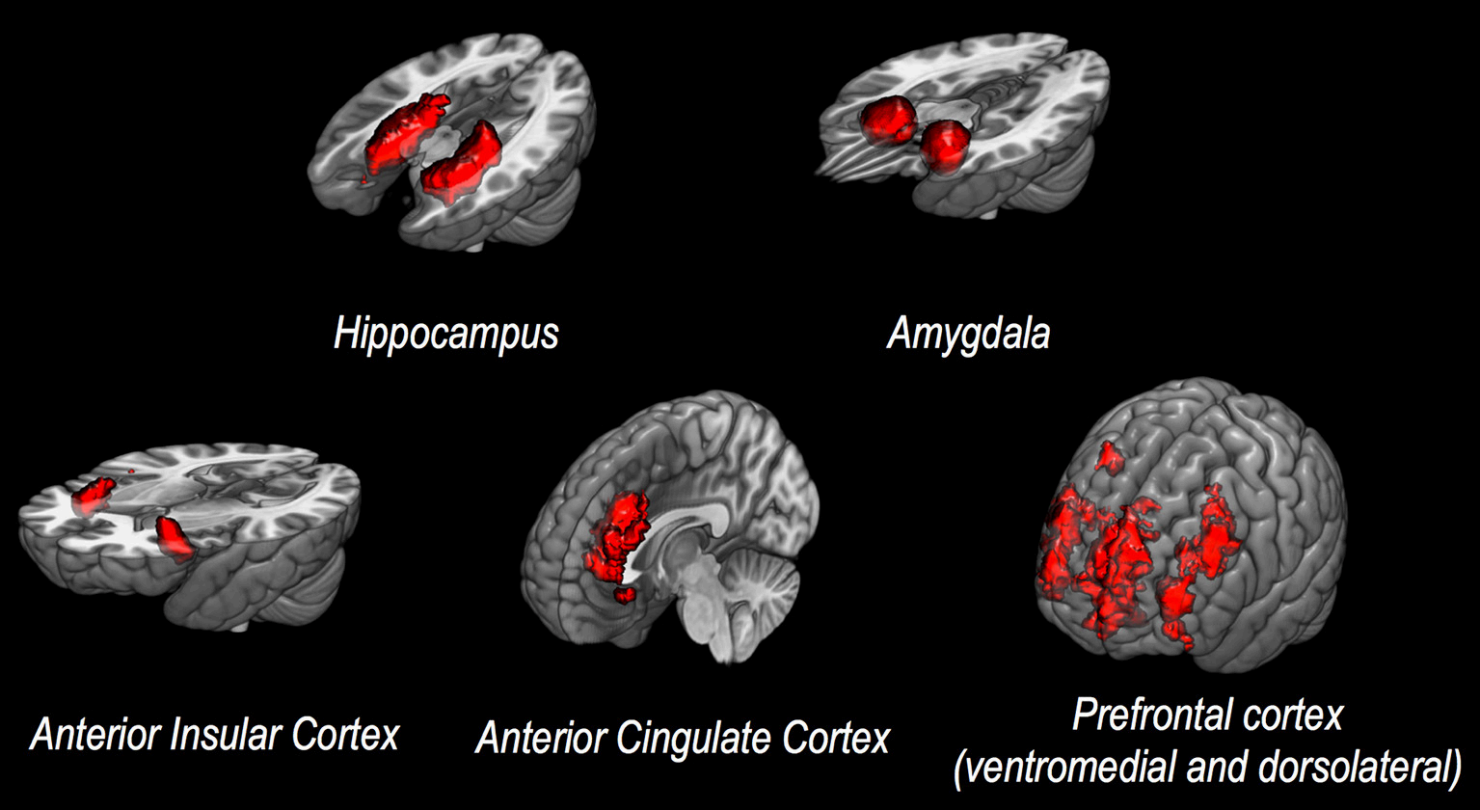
Discussed neuroanatomical structures. This figure presents neuroanatomical target regions frequently investigated via structural or functional MRI in studies assessing potential neurobiological correlates of vulnerability to stress and/or resilience.
